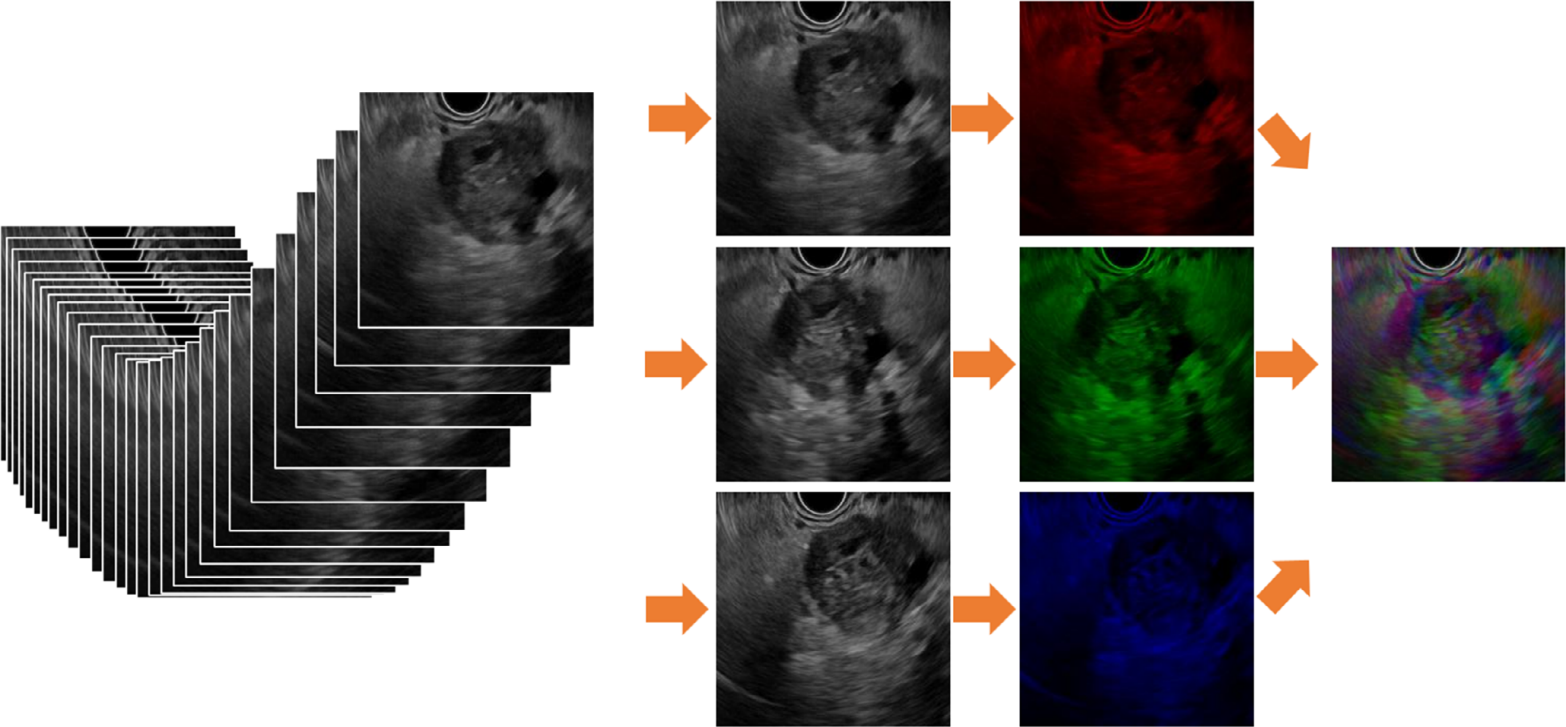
Quasi‐RGB images. Three grayscale images were randomly selected from endoscopic ultrasonography frames to create a quasi‐RGB image. A single quasi‐RGB image carries temporal data and contributes to data augmentation.
